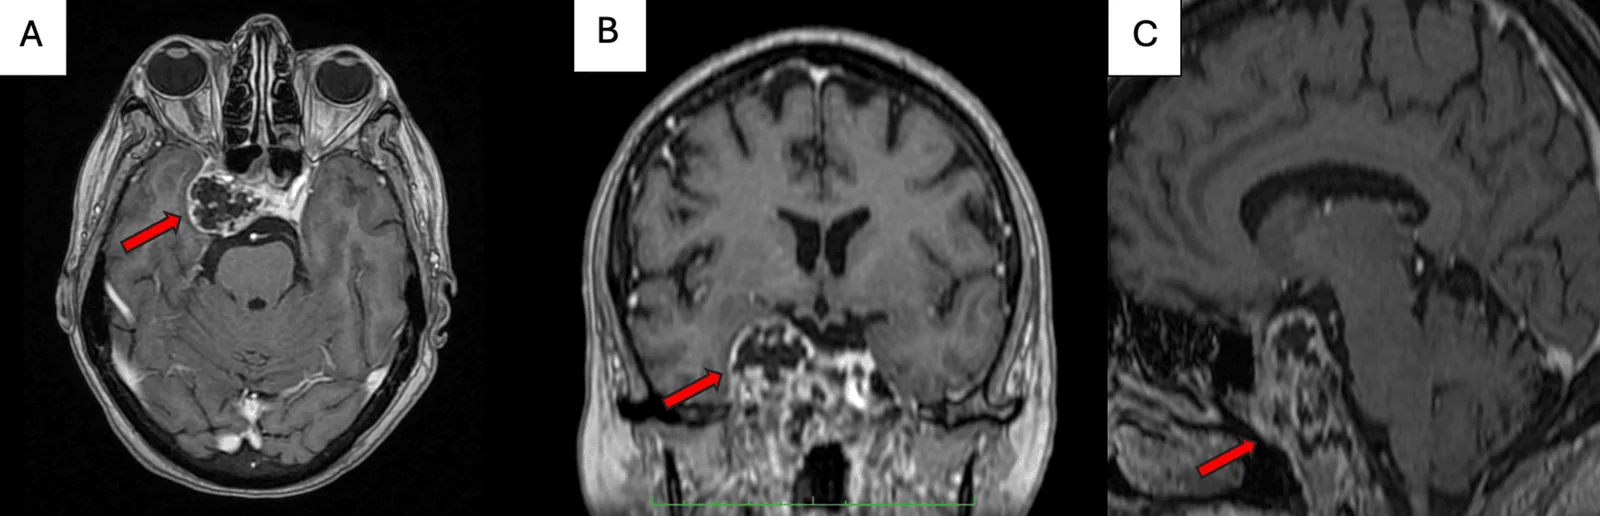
MRI of the clival lesion in October 2024 MRI obtained in October 2024. (A) Axial, (B) coronal, and (C) sagittal contrast-enhanced T1-weighted images demonstrating an expansile heterogeneous lesion centered in the clivus, with extension into the sphenoid sinus and right cavernous sinus. Red arrows indicate the lesion and its extension.
